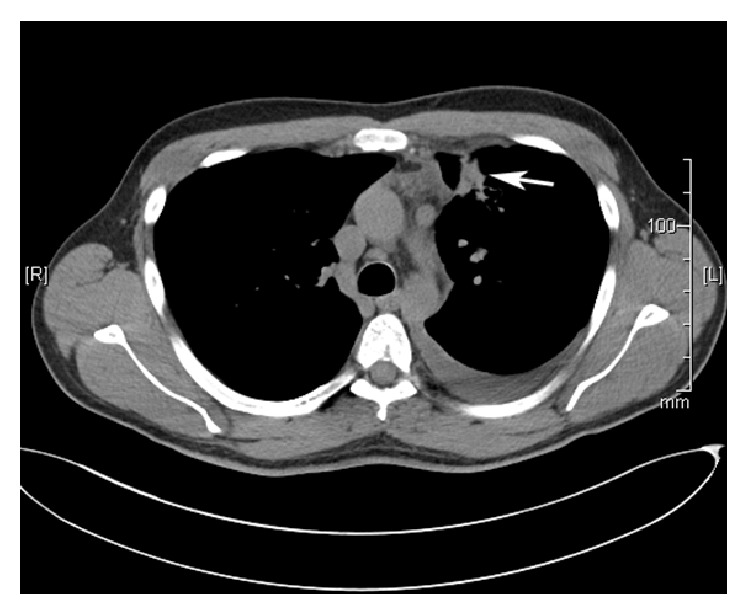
Chest CT showing the mass/area of consolidation in the left upper lobe (arrow).
